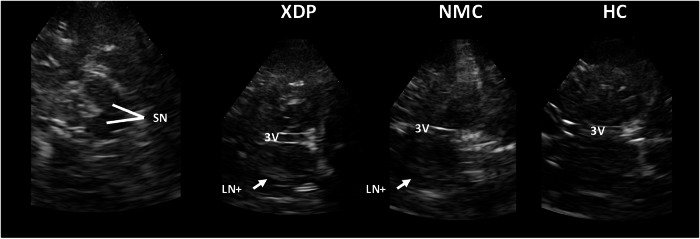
Transcranial sonography. Transcranial sonography imaging of the substantia nigra (SN), the third ventricle (3V), the lentiform nucleus with (LN+) or without hyperechogenicity (LN−) in patients with X-linked dystonia-parkinsonism (XDP), non-manifesting carriers (NMC), and healthy controls (HC).
